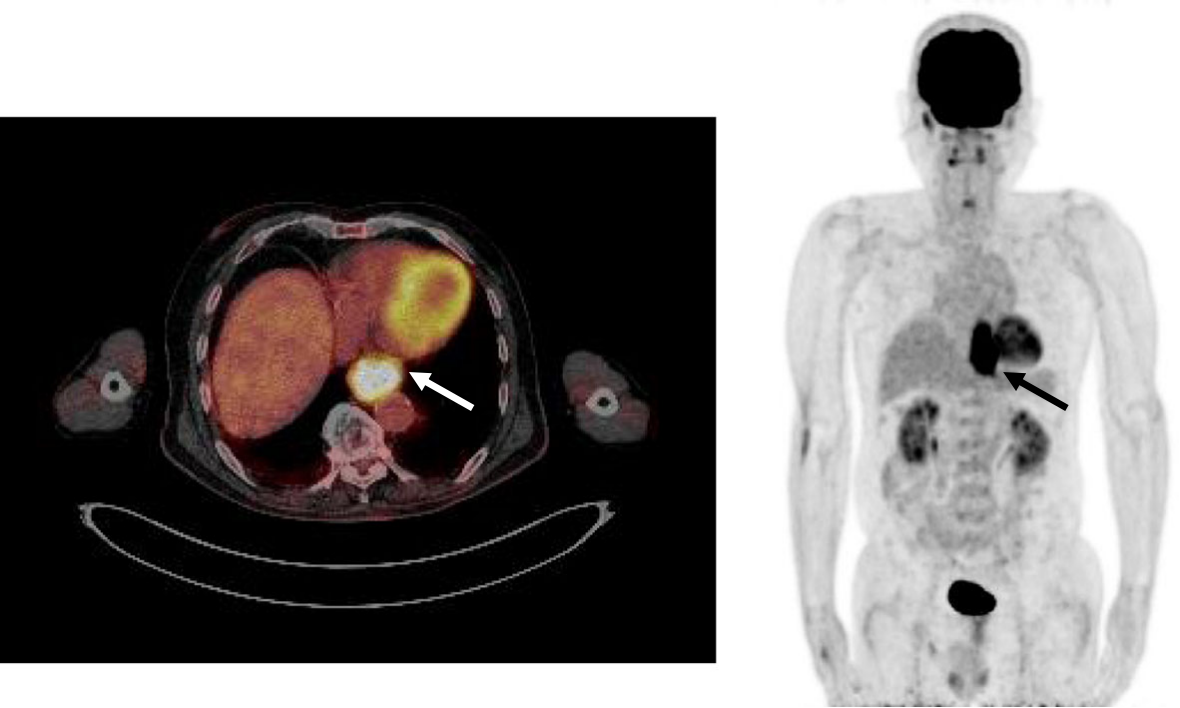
Positron emission tomography (PET)-CT revealed accumulation of fluorine-18-2-fluoro-2-deoxy-d-glucose (FDG) in the lower thoracic esophagus, with a standardized uptake value of 8.8 (arrow)
